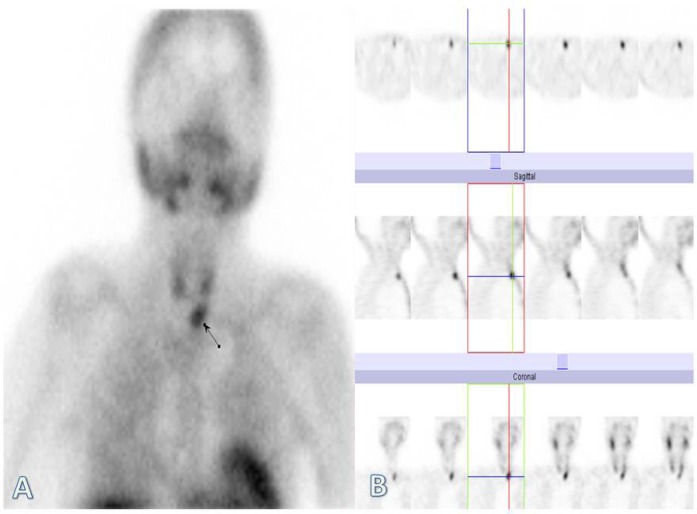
Tc-99m sestamibi scintigraphy of the parathyroid. The early phase images on planar (A) and SPECT views (B), showed radiotracer uptake in the thyroid region, as well as a focal zone of increased tracer uptake in the left lower part of the neck inferior to the thyroid
